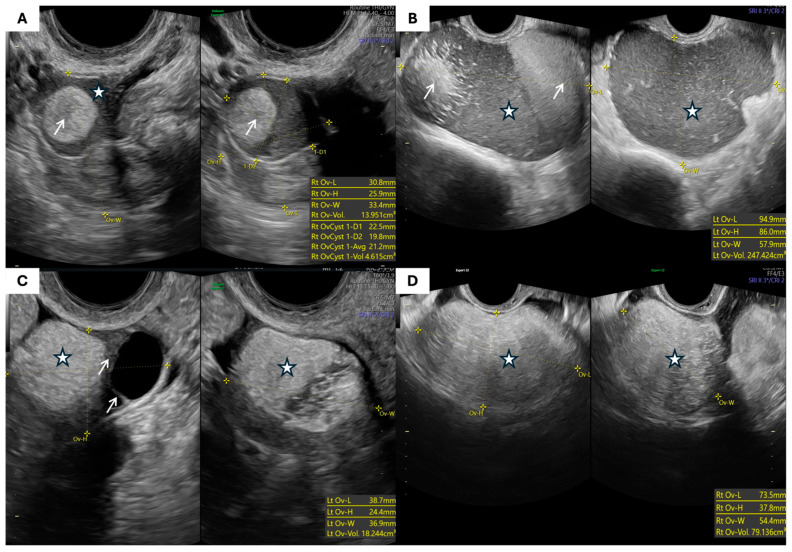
Ultrasonographic appearances of mature cystic teratomas: (A,B) Multilocular solid ovarian teratoma (arrows point to hyperechoic areas and solid tissue components; asterisks mark cystic components). (C) Multilocular solid ovarian teratoma (arrows point to septations; asterisk indicates hyperechoic solid component). (D) Unilocular solid ovarian teratoma (asterisk indicates hyperechoic solid component).
